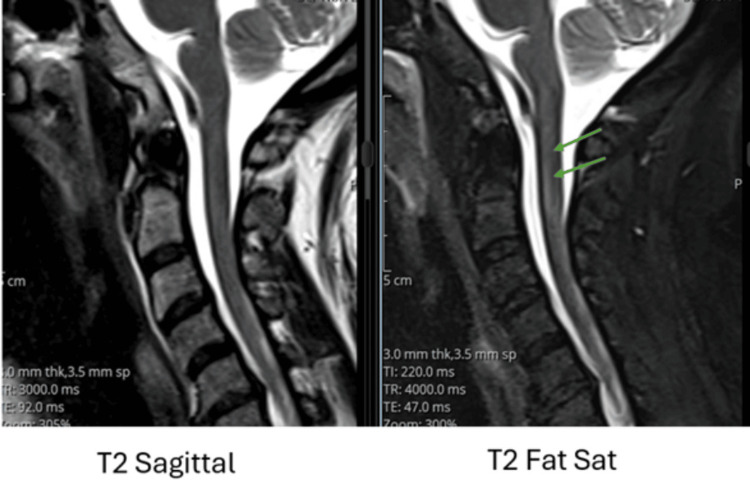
Initial spinal cord hyperintensity on magnetic resonance imaging (MRI) of the cervical spine Sagittal T2-weighted and T2 fat-saturated MRI sequences of the cervical spine show a longitudinal linear hyperintense signal in the posterior spinal cord from C2 to C5 (green arrows), suggestive of early ischemic or inflammatory changes.
